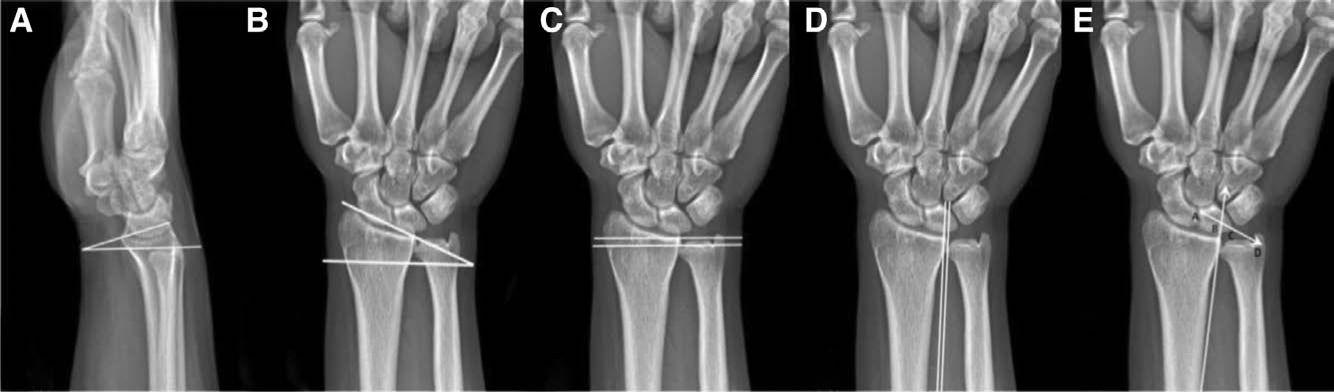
X-ray parameters measured in this study. (A) Volar tilt; (B) radial inclination; (C) ulnar variance; (D) DRUJ interval; (E) coronal plane displacement. DRUJ = distal radioulnar joint.
